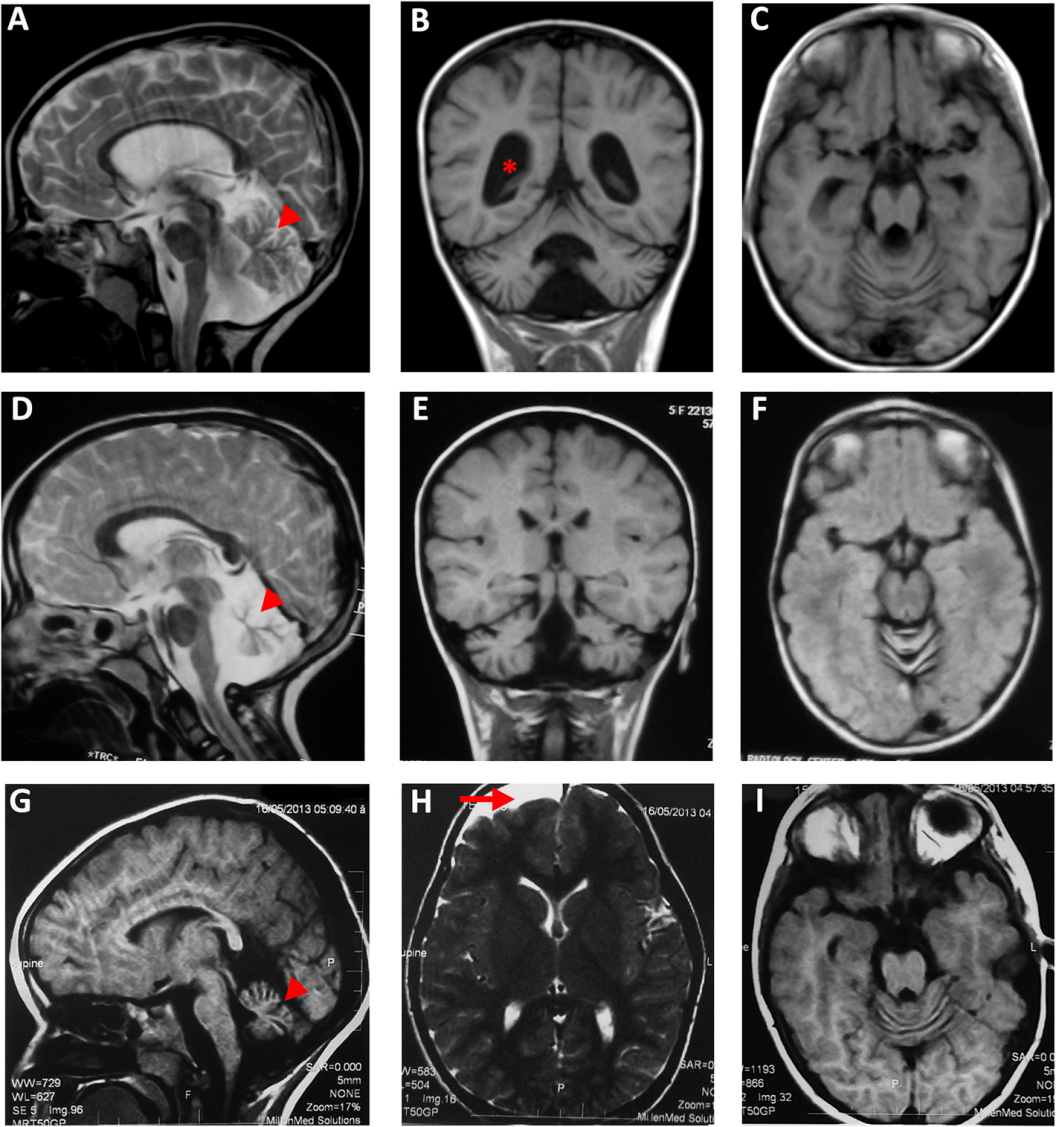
Brain MRI scans from families CIE17, CIE21 and CIE12. a-c MRIs of case CIE17-A1 (*MFSD8* mutation) at 3 years. a Midline Sagittal T2-weighted image demonstrating enlarged cerebellar folia (*arrow head*) and thin corpus callosum. Ventricular system dilatation (*star*) is visible on coronal T1 image (b). d-f MRIs of patient CIE-21-A1 (*KIF1A* mutation) at 5 years showing cerebellar atrophy (*arrowhead*) on midline sagittal T2 (d), coronal T1 (e) and axial T1 (f) images. g-i MRIs of patient CIE12-A2 (*MOCS2* mutation) with evidence of cerebellar vermis atrophy (*arrowhead*) on sagittal T1 image (g) and Right frontal arachnoid cyst (*red arrow*) visible on axial T2 scan (h)
